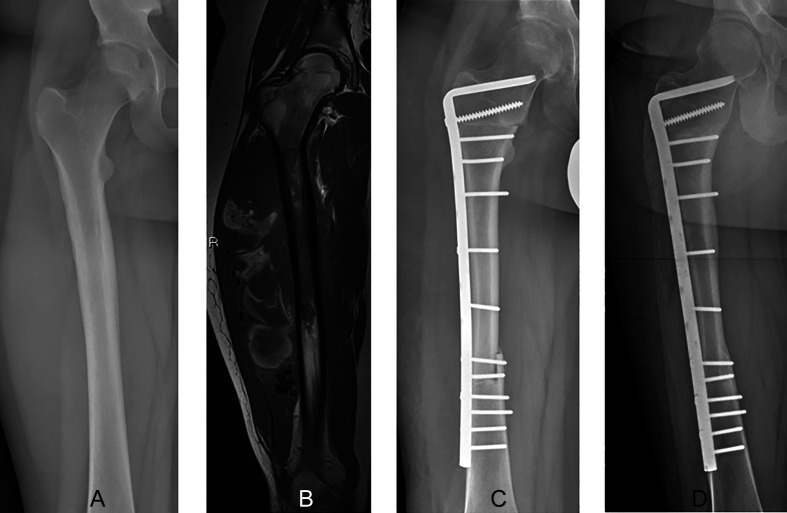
A 16-year-old boy with a diagnosis of Ewing sarcoma of the right femur. Preoperative anteroposterior radiographic view (A) showing a lytic lesion with periosteal reaction at the proximal diaphyseal region of the right femur. Coronal-T1 (B) magnetic resonance image shows tumor extension and a large extraosseous mass at the proximal diaphysis without involvement of the meta-epiphyseal region of the femur. Radiographs showing intercalary reconstruction of the femur using a massive bone allograft at the three-month (C) and 8-year (D) follow-up, showing consolidation and integration of the massive bone allograft. The massive bone allograft was able to be incorporated into the host bone despite the absence of the vascularized fibula graft as the resection was less than 15 cm.
